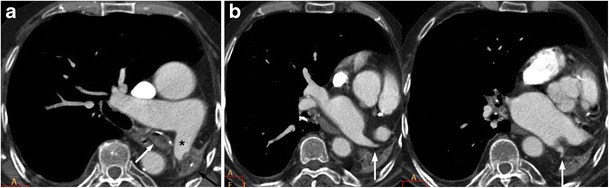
An 83-year-old male who had pulmonary TB in infancy came to our hospital for weakness and paresthesia of both hands. (a) On contrast-enhanced multislice CT, total left lung destruction with calcifications (black arrow) in the remnant lung, occupation of the left main bronchus (white arrow) and decreased diameter of the left main pulmonary artery (asterisk) are visualised. (b) The diameter of the ipsilateral superior and inferior pulmonary veins is also decreased (arrows)
